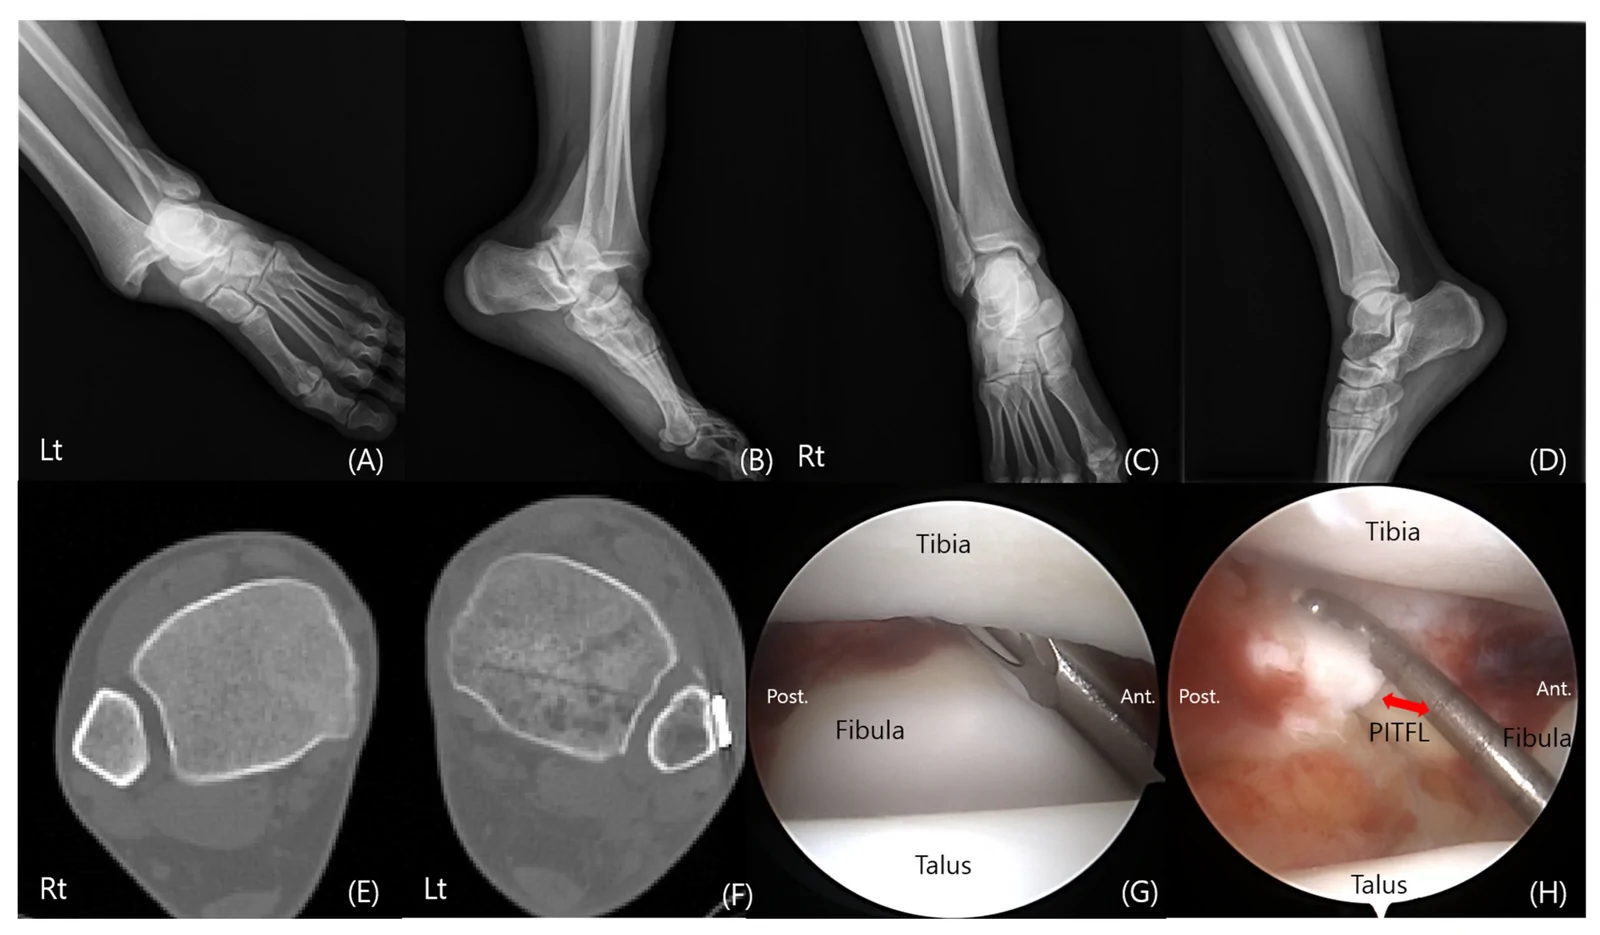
Representative images of Patient 1. (A,B) Initial radiographs demonstrating a left Lauge-Hansen SER type IV ankle fracture-dislocation with lateral and posterior malleolar fractures: (A) anteroposterior view and (B) lateral view. (C,D) Initial radiographs of the uninjured contralateral right ankle: (C) anteroposterior view and (D) lateral view. (E,F) One-year postoperative axial CT images obtained 1 cm proximal to the tibial plafond: (E) uninjured right ankle and (F) injured left ankle. (G) Arthroscopic image showing AITFL rupture, with widening of the anterior syndesmotic gap, permitting insertion of a 3 mm shaver tip. (H) Arthroscopic image showing PITFL rupture at its tibial insertion. The red arrow indicates the course of the PITFL. SER, supination–external rotation; CT, computed tomography; AITFL, anterior inferior tibiofibular ligament; PITFL, posterior inferior tibiofibular ligament; Lt, left; Rt, right; Ant., anterior; Post., posterior.
